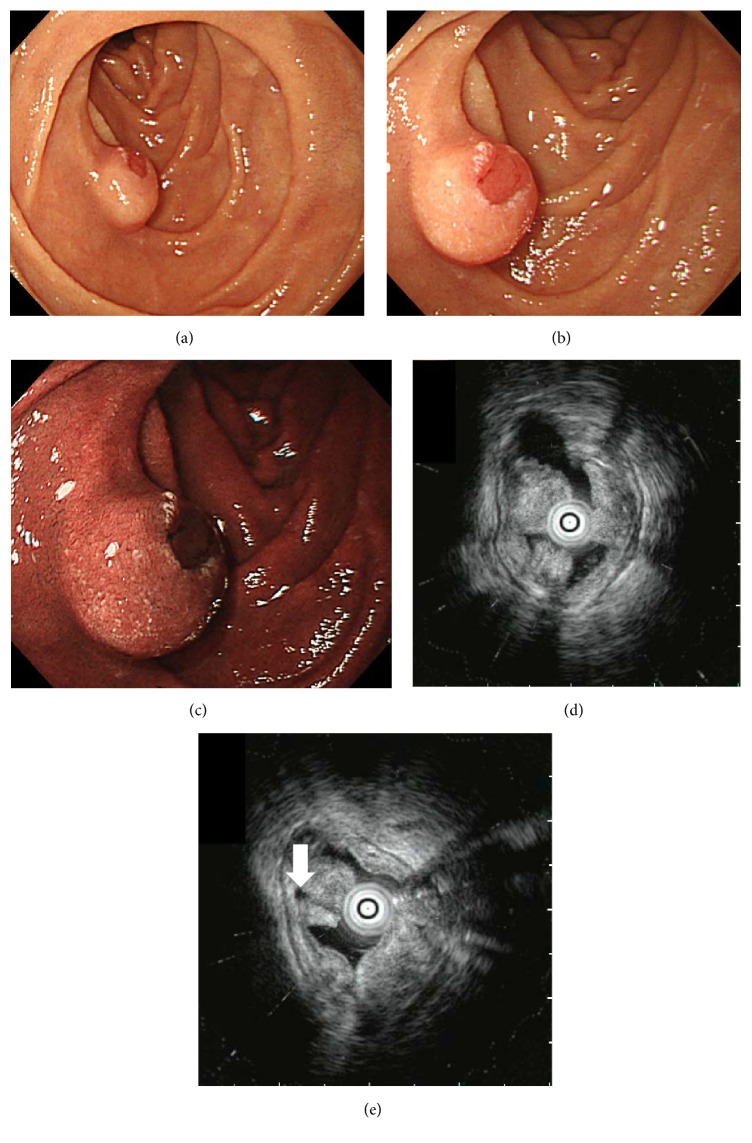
Endoscopic images. Esophagogastroduodenoscopy shows a solitary submucosal tumor in the duodenal second portion. The top of the tumor is slightly depressed, showing a reddish color on white light imaging (a, b) and a brownish color on narrow band imaging (c). Endoscopic ultrasonography reveals that the hypoechoic mass is mainly confined to the mucosal layer (d). A small cystic area is also seen (white arrow) (e).
